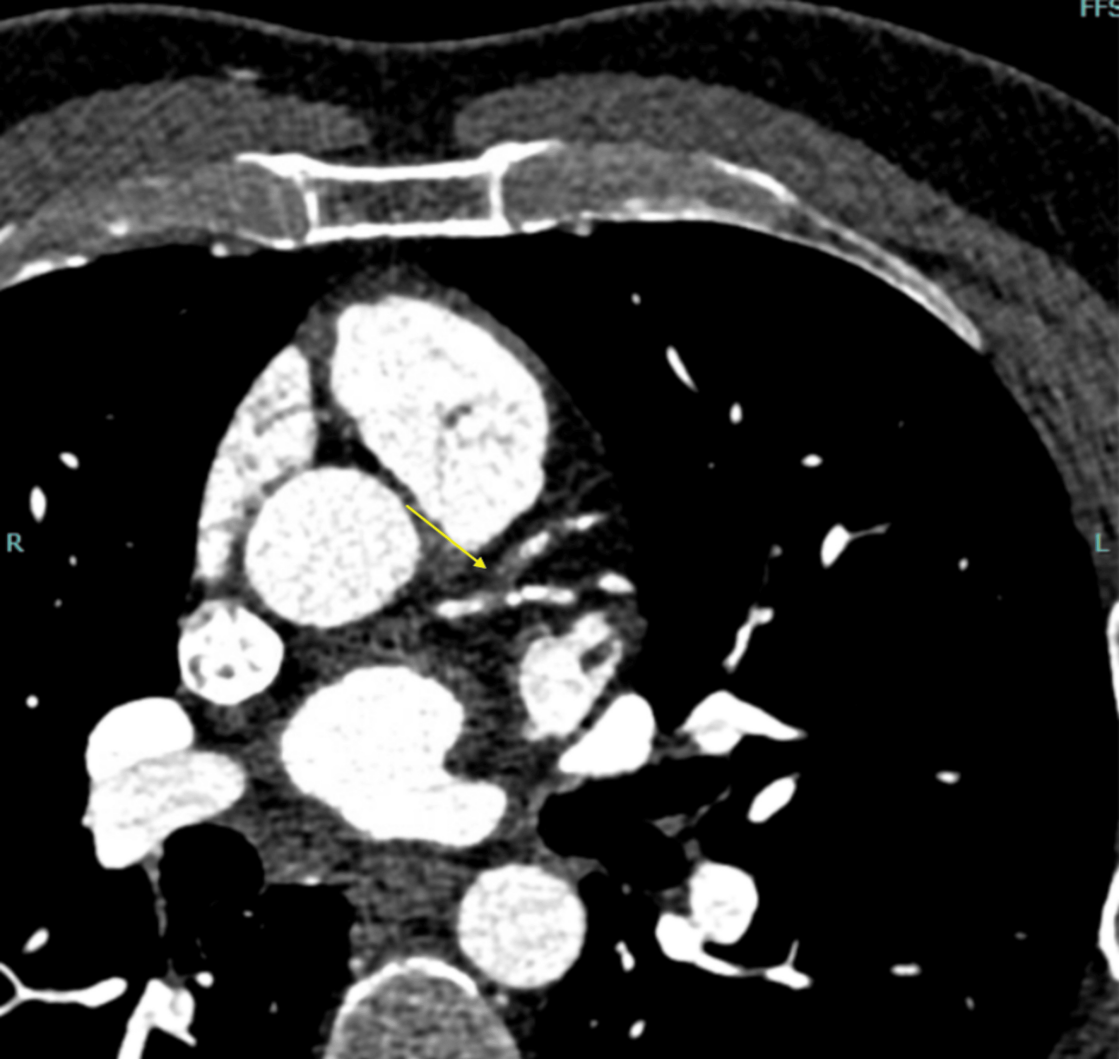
CTA demonstrating stenosis of the LAD artery (yellow arrow) CTA: CT angiogram; LAD: Left anterior descending
